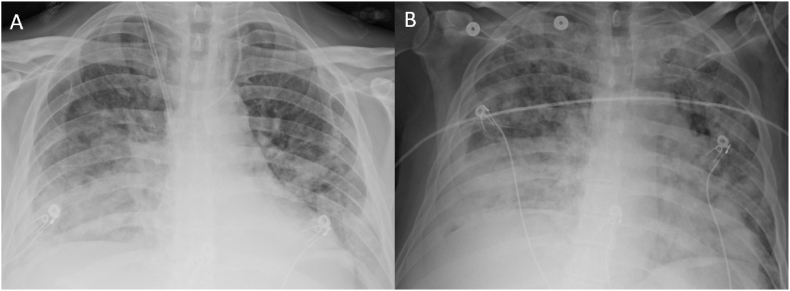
(A) Chest radiograph obtained on day +26 of admission for fever showed new bilateral airspace opacities. (B) Chest radiograph from day +32 shows interval progression of airspace disease.
